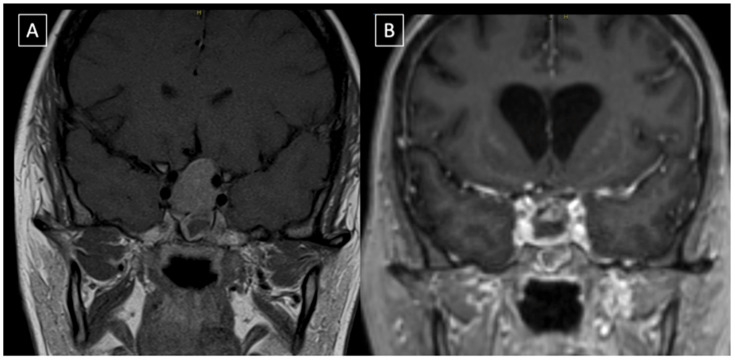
Coronal reconstruction of the pre-op (A) and post-op (B) post gadolinium T1-weighted images. The post-operative MRI confirms that optic chiasm has been decompressed and gross total resection obtained. There is evidence of minimally enhancing intrasellar material that is likely gel foam used for skull base reconstruction.
